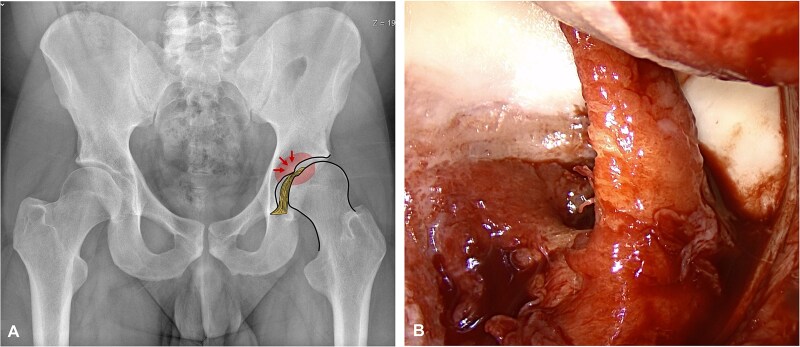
(A) The fovea capitis in this male patient with developmental dysplasia of the hip and coxa valga is located more superiorly and decentralized outside the acetabular fossa on the anteroposterior pelvic radiograph. (B) The intraoperative documentation from surgical hip dislocation shows a degenerated ligamentum teres and associated lesions on the acetabular fossa cartilage in this patient.
